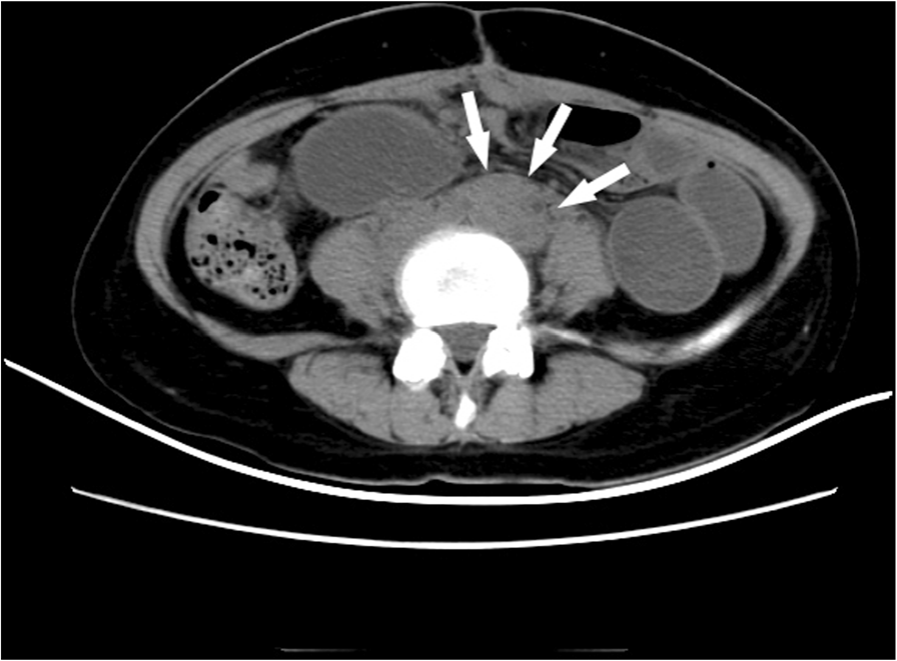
Nonenhanced abdominal computed tomography scan. The scan revealed para-aortic lymph node enlargement measuring approximately 4 cm in diameter (arrows).
